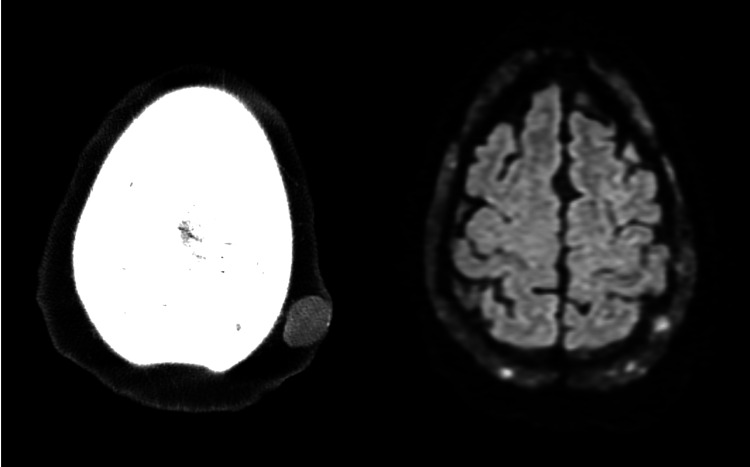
CT of the head revealing left parietal nonenhancing solid nodule (left image) and lytic lesions (right image) CT: computed tomography
